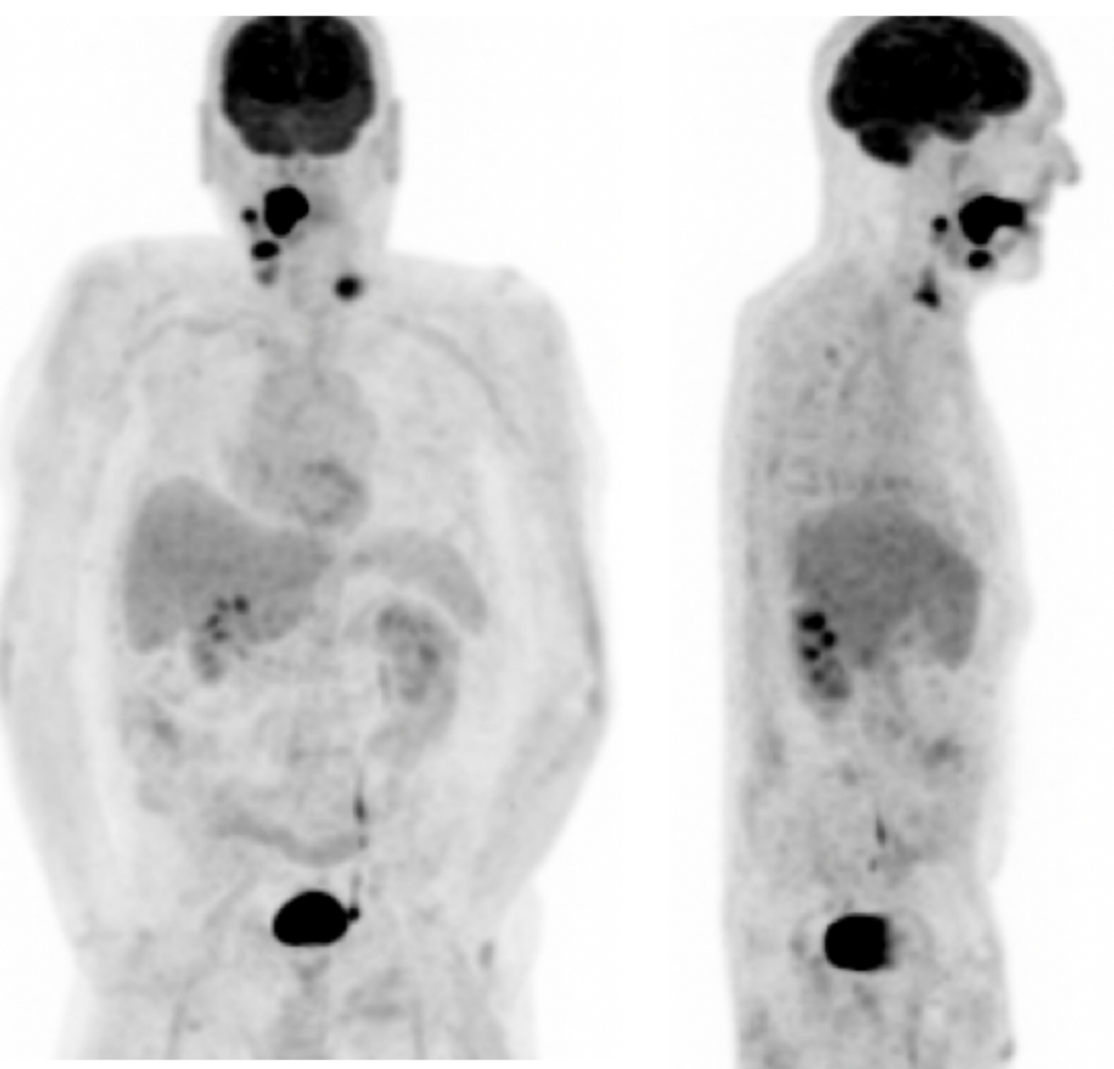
Whole body PET/CT scan showing increased metabolism in the tongue and nearby locations along with supraclavicular lymph node.
